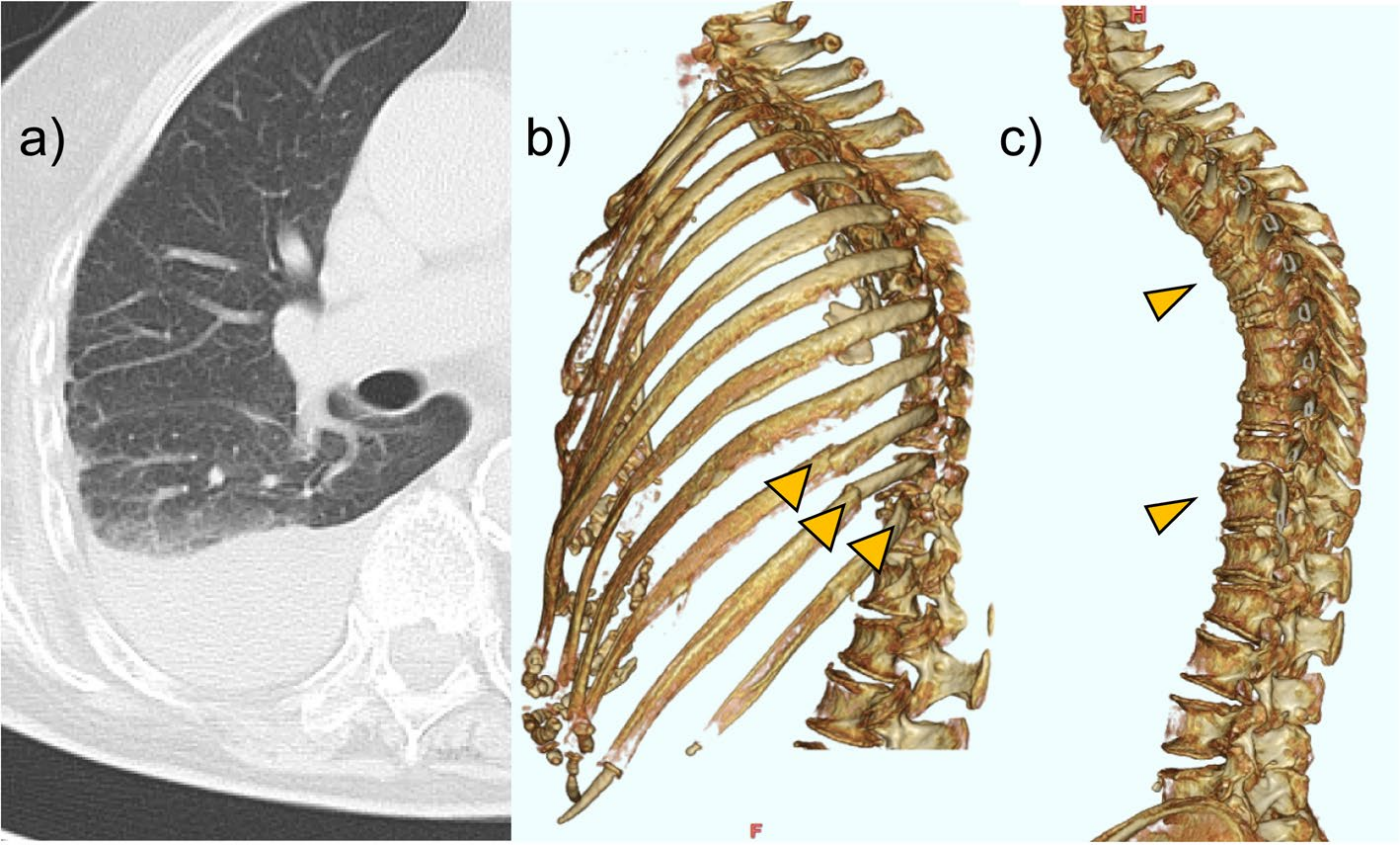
CT images obtained during transport. a Right hemothorax, b fractures of the 9th–11th ribs on the left side, and c multiple thoracic vertebral fractures. CT, computed tomography
